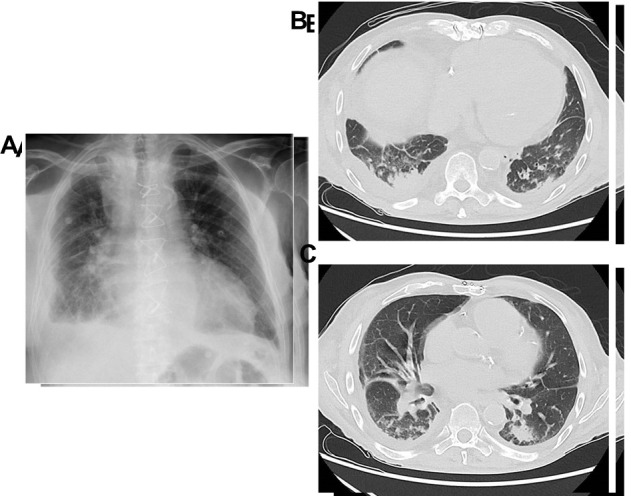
Elderly patient with a high clinical suspicion of pneumonia. Because of overlapping pulmonary oedema and effusion, X-ray (A) was non-conclusive. In a subsequently performed CT scan, however, small consolidations in (B, C) right lower lobe are visible.
